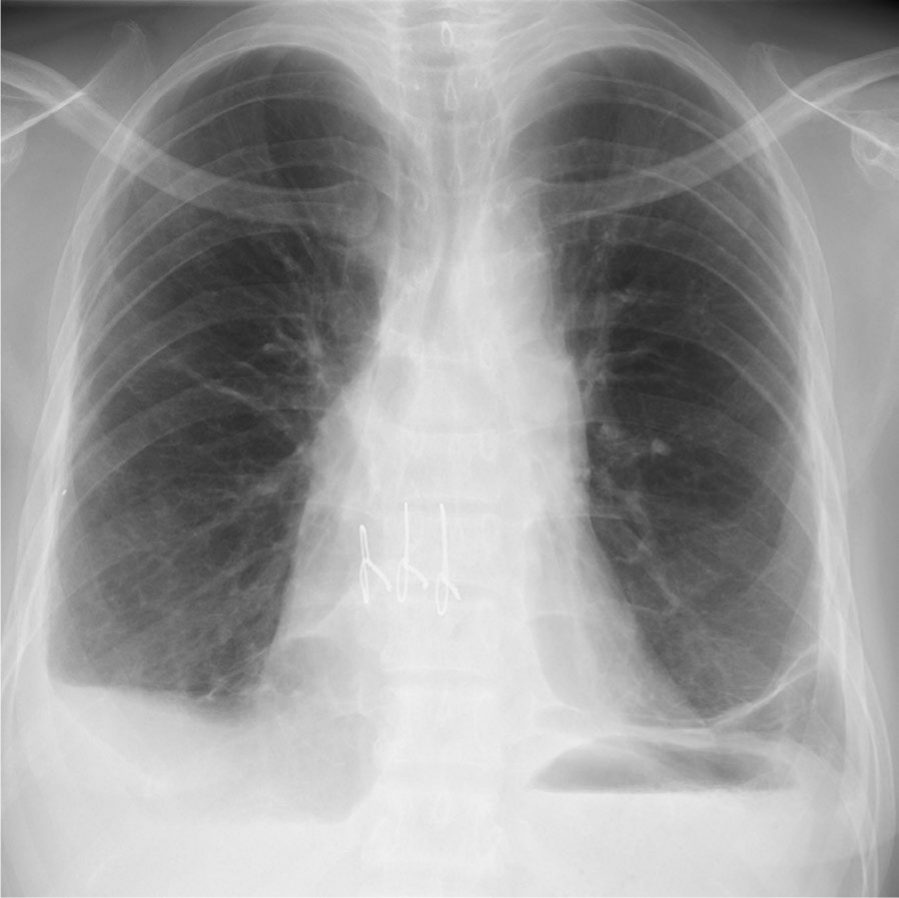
Chest radiograph just before discharge showing fully expanded clear lung fields
